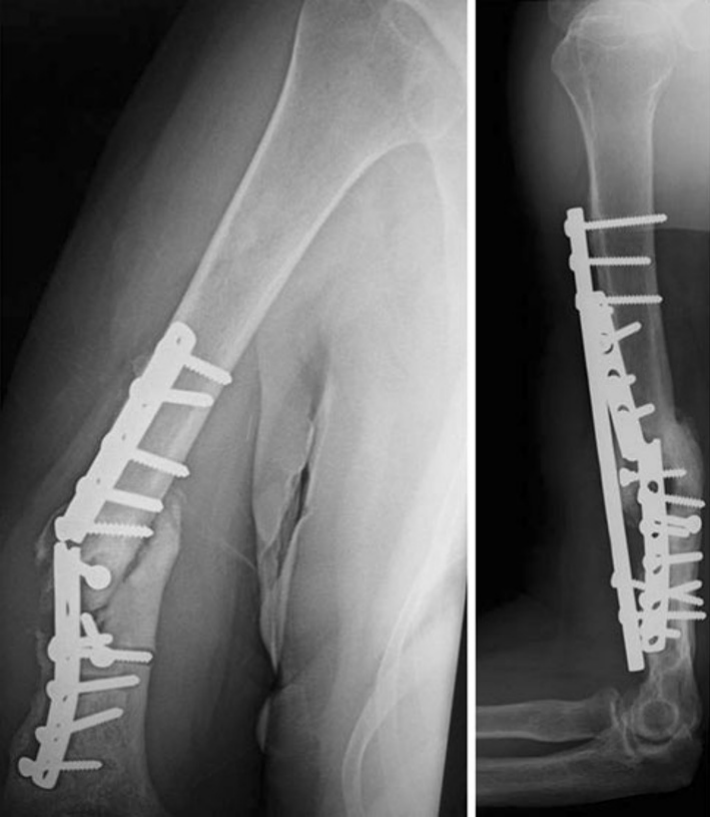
X-ray images demonstrate atrophic nonunion with previous instability signs (*left*) healed 6 weeks after the surgery (*right*)
